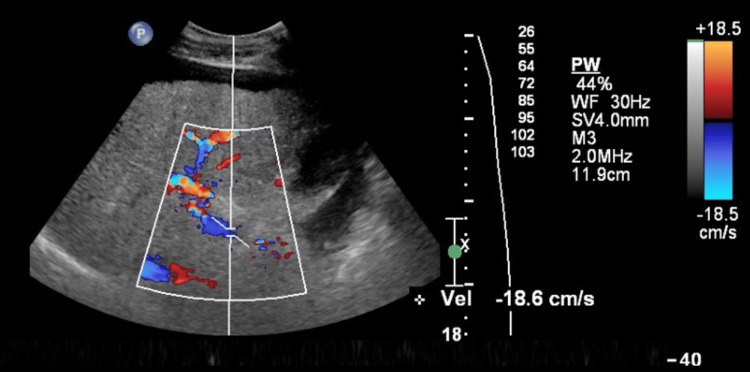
Oblique view of portal vein revealing cirrhosis with reversal of portal venous flow and portal hypertension by color Doppler
